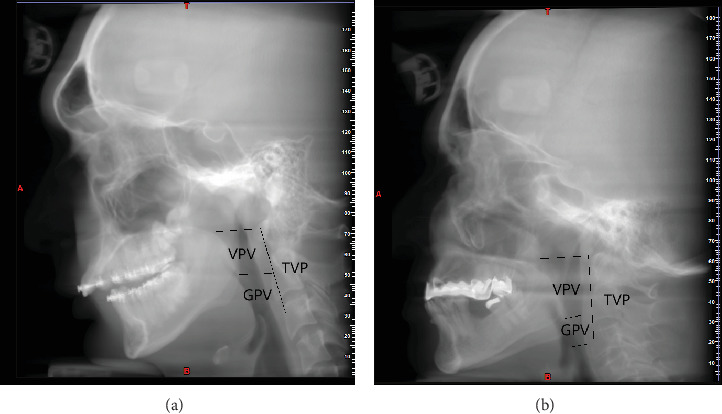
TVP, VPV, and GPV of Class II (A) and Class III (B) patients in a CBCT image.
